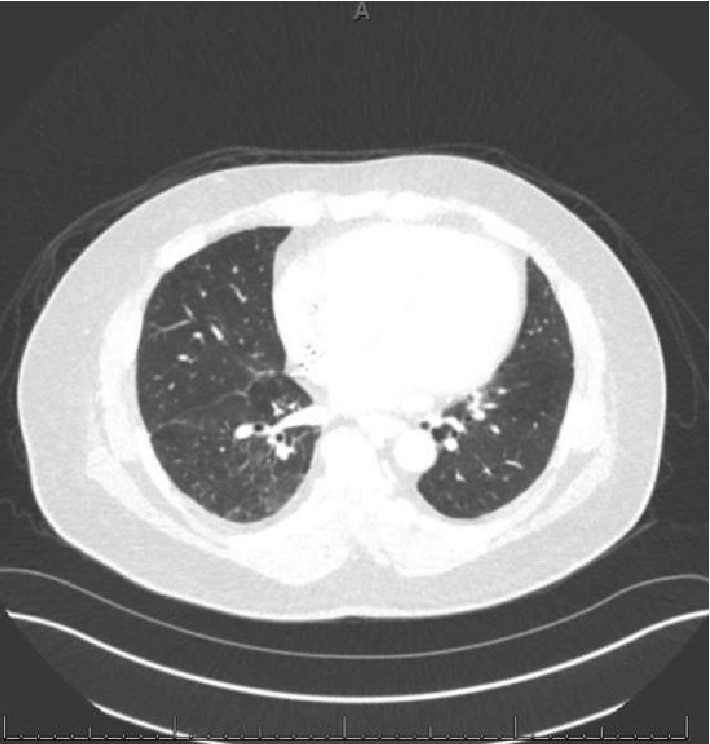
CT chest w/ contrast, lung window, showing lung findings post ~4 months of initial pneumonitis findings: mild emphysematous changes noted.
